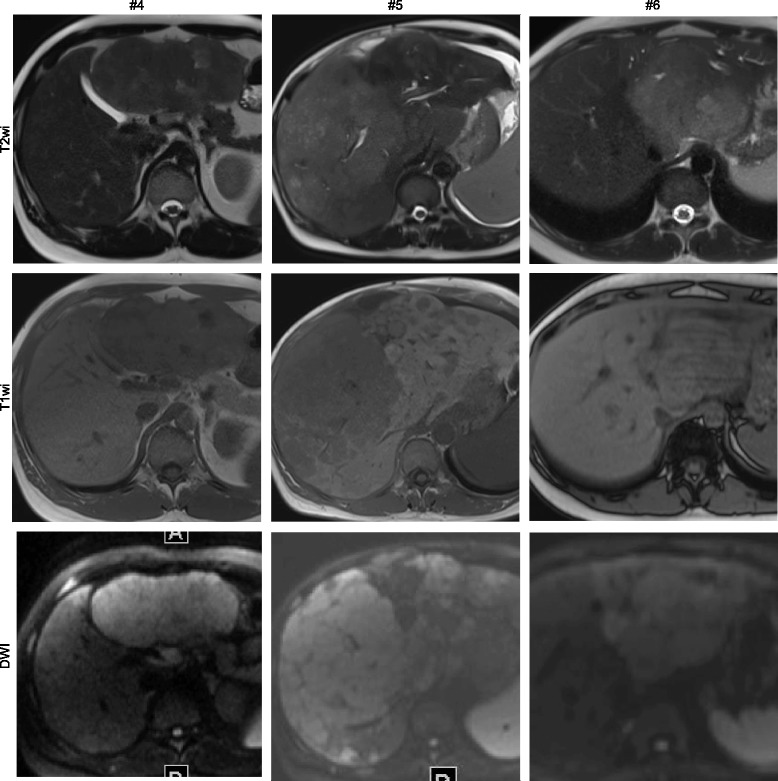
Image panel of patients #4, #5, and #6 displaying representative sections through individual fibrolamellar carcinoma in non-contrast enhanced imaging techniques including T2 weighted imaging (T2wi), T1 weighted imaging (T1wi), and diffusion weighted imaging using high b-values (DWI)
